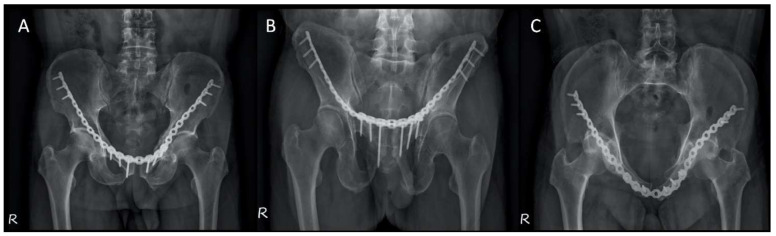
Postoperative X-ray films of the pelvis. (A) AP view, (B) outlet view, and (C) inlet view.
